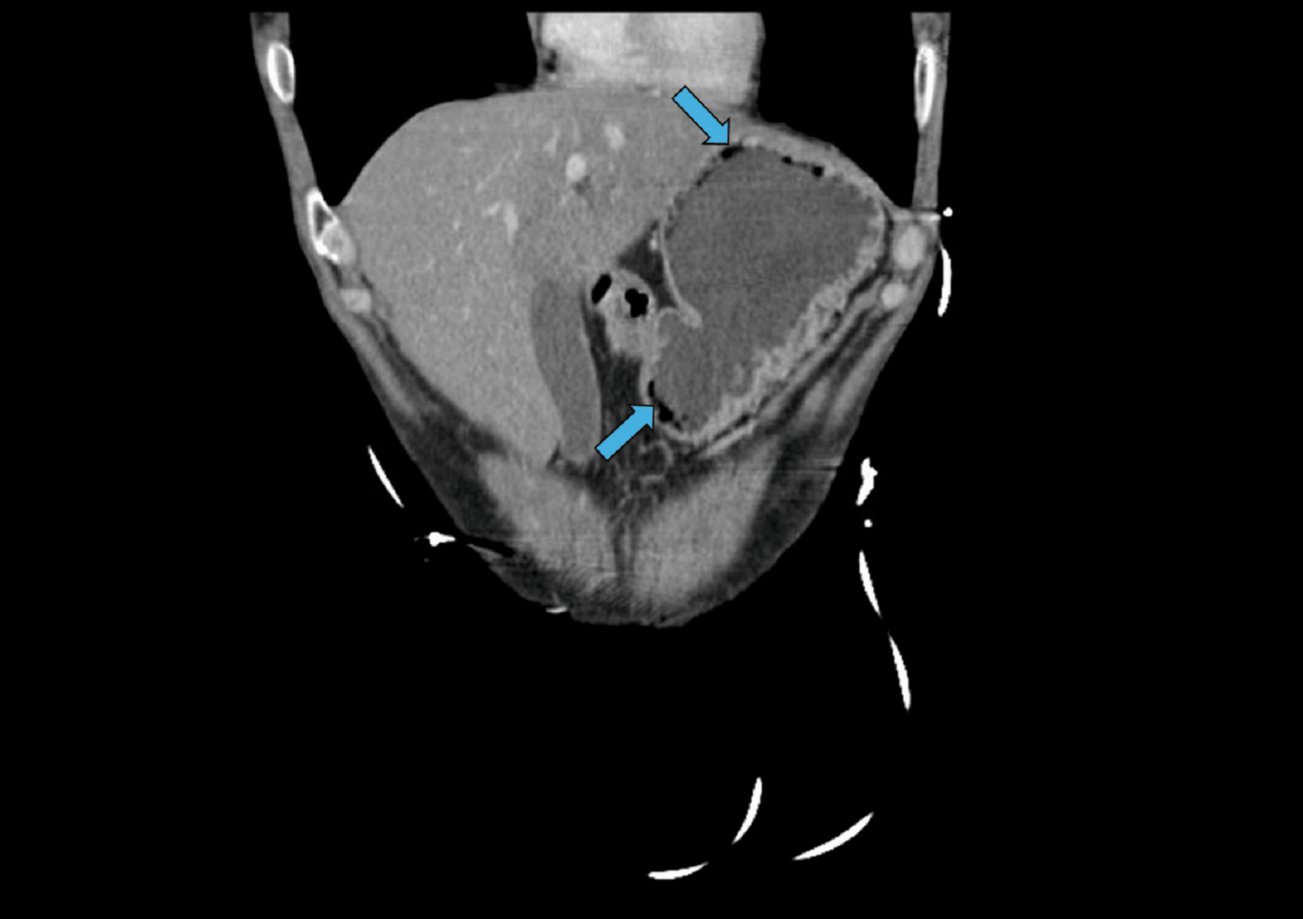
Computed tomography scan (coronal view) Blue arrows indicate the presence of gas within the stomach wall.
